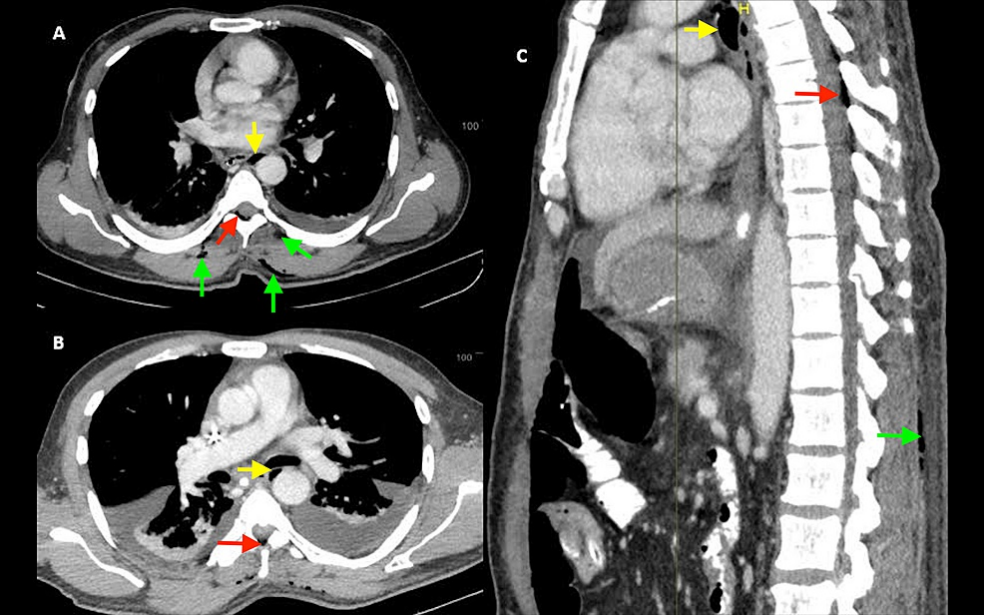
CT scan of the chest The scan images in axial (A, B panels) and sagittal (C panel) planes depicting air in the epidural space (red arrows), subcutaneous tissue intermuscular plane (green arrows), and posterior mediastinum (yellow arrows) CT: computed tomography
